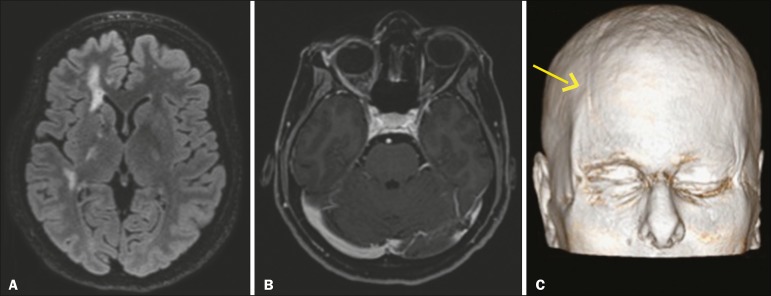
Female patient being followed for convulsions. A: Axial MRI
